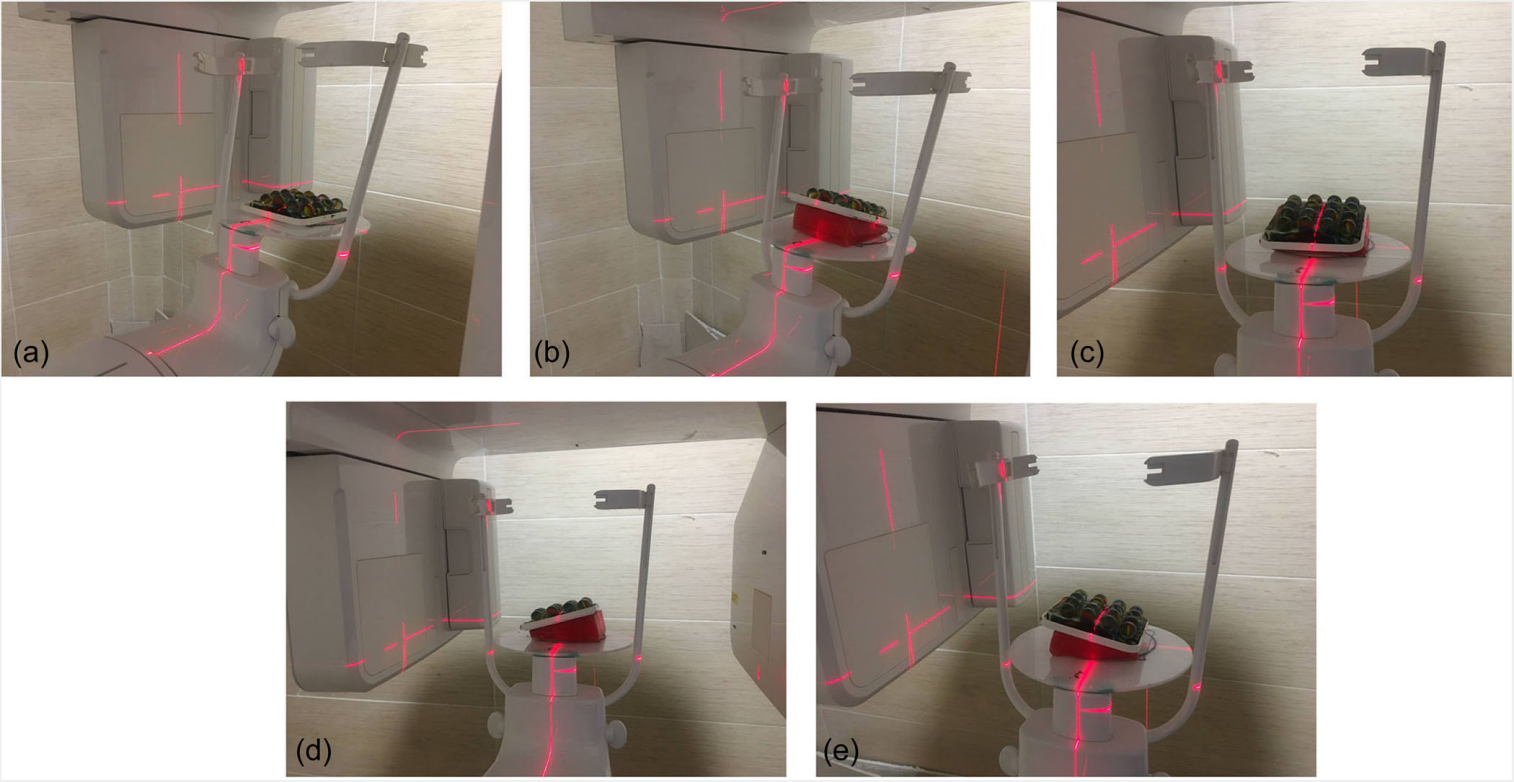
Different scaning positions and stabilization on a flat support on the XMIND Trium CBCT scanner. (a) neutral, (b) 20° tilt to up, (c) 20° tilt to down, (d) 20° tilt to right, (e) 20° tilt to left. CBCT, Cone‐beam computed tomography.
